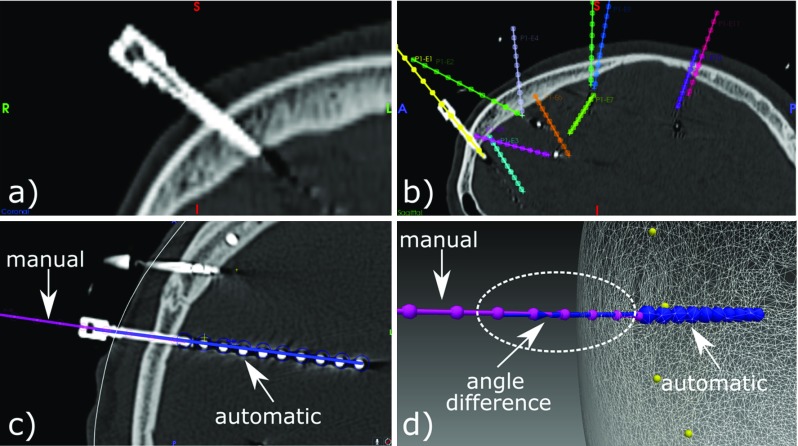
Bolt angles. a Bolt from post-CT image and b manual identification of the direction along bolts by a clinical scientist. c, d (Inconspicuous) comparison of manual (pink) and automatic identification (rigid electrode shown in blue) of bolt direction of an outlier case with angle difference of $$\theta _{\mathrm{error}}=5.73^{\circ }$$ and displacement error at the tip of $$d_{\mathrm{error}}=4.79$$ mm
